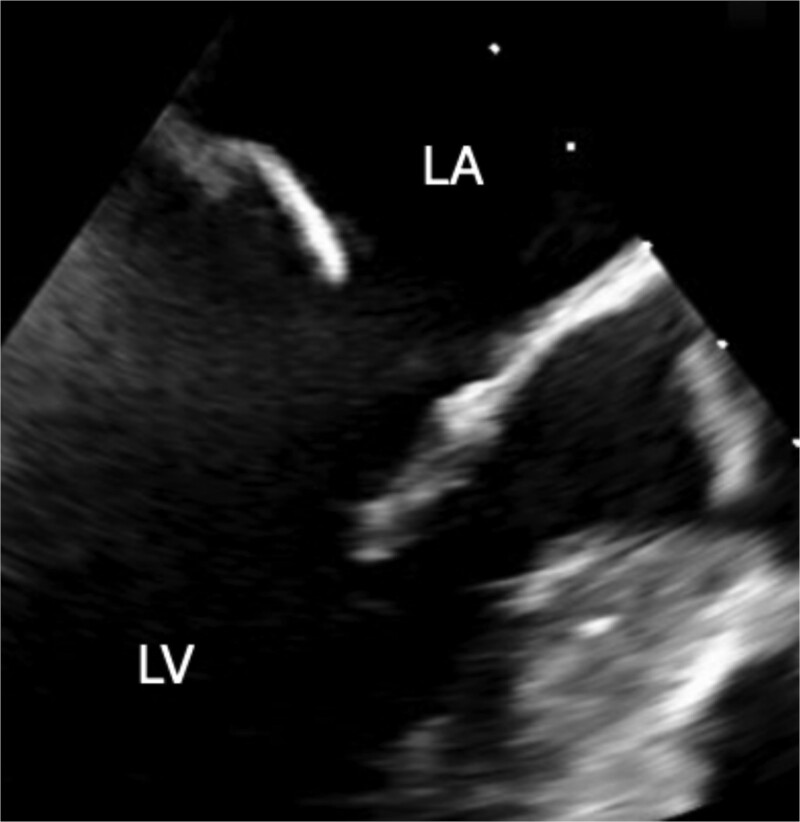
Preoperative transesophageal echocardiography showing disappearance of the mass.
